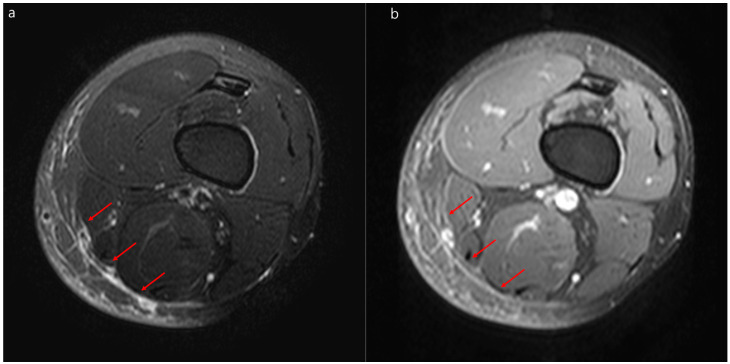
Representative magnetic resonance images of severe cellulitis. Severe cellulitis of the left thigh in a 44-year-old man. (a) Fat-suppressed axial T2-weighted magnetic resonance image and a contrast-enhanced magnetic resonance image (b) showing localized hyperintensity within the deep peripheral fascia (arrows) with enhancement in the posteromedial thigh.
